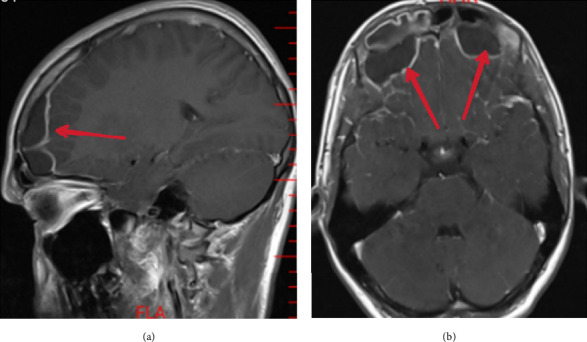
The brain MRI of the patient. (a) A sagittal T1-weighted view of the brain, showing 1.1 cm collection of fluids in the frontal sinus. (b) An axial T1-weighted view of the brain which shows bilateral frontal epidural mucocele. Note the bigger size of the collection on the left side. The frontal sinus collection extends toward the cranium which is seen as an epidural mucopyocele. The dura appears intact, but the posterior wall of the frontal sinus and the corresponding epidural space were damaged, both of which were confirmed by viewing the CT scan performed at the patient's hometown hospital and noted during the operation.
